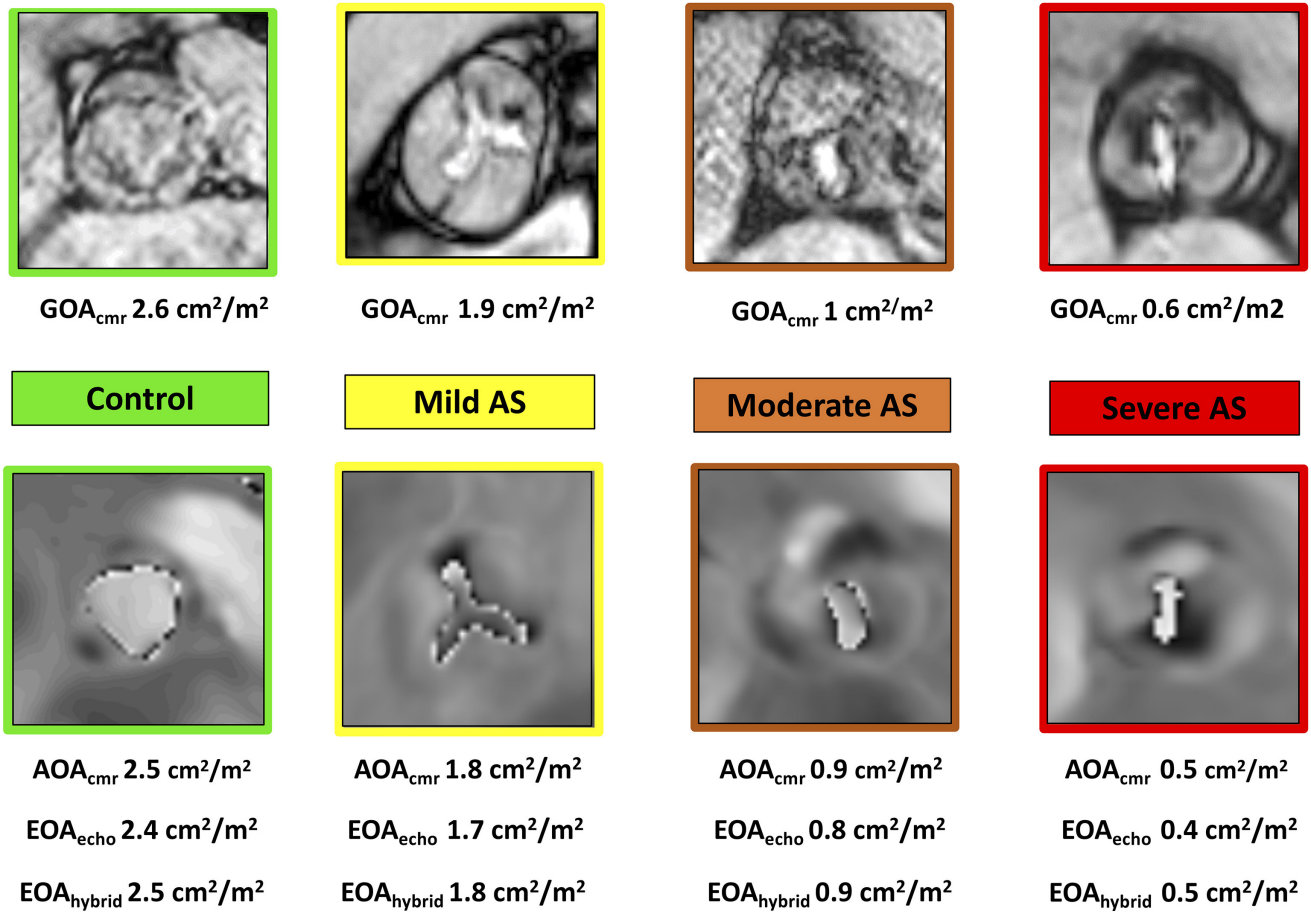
Case examples of normal aortic valve area (green), mild (yellow), moderate (orange), and severe (red) aortic stenosis measured with GOA planimetry (top row) by cine CMR and AOA planimetry by fixed low-VENC phase contrast CMR (bottom row). All measurements have been indexed to body surface area and reported as cm^2^/m^2^. AOA, aliased orifice area; EOA, effective orifice area; CMR, cardiovascular magnetic resonance; GOA, geometric orifice area; echo, echocardiography; hybrid, combined echo-CMR assessment.
